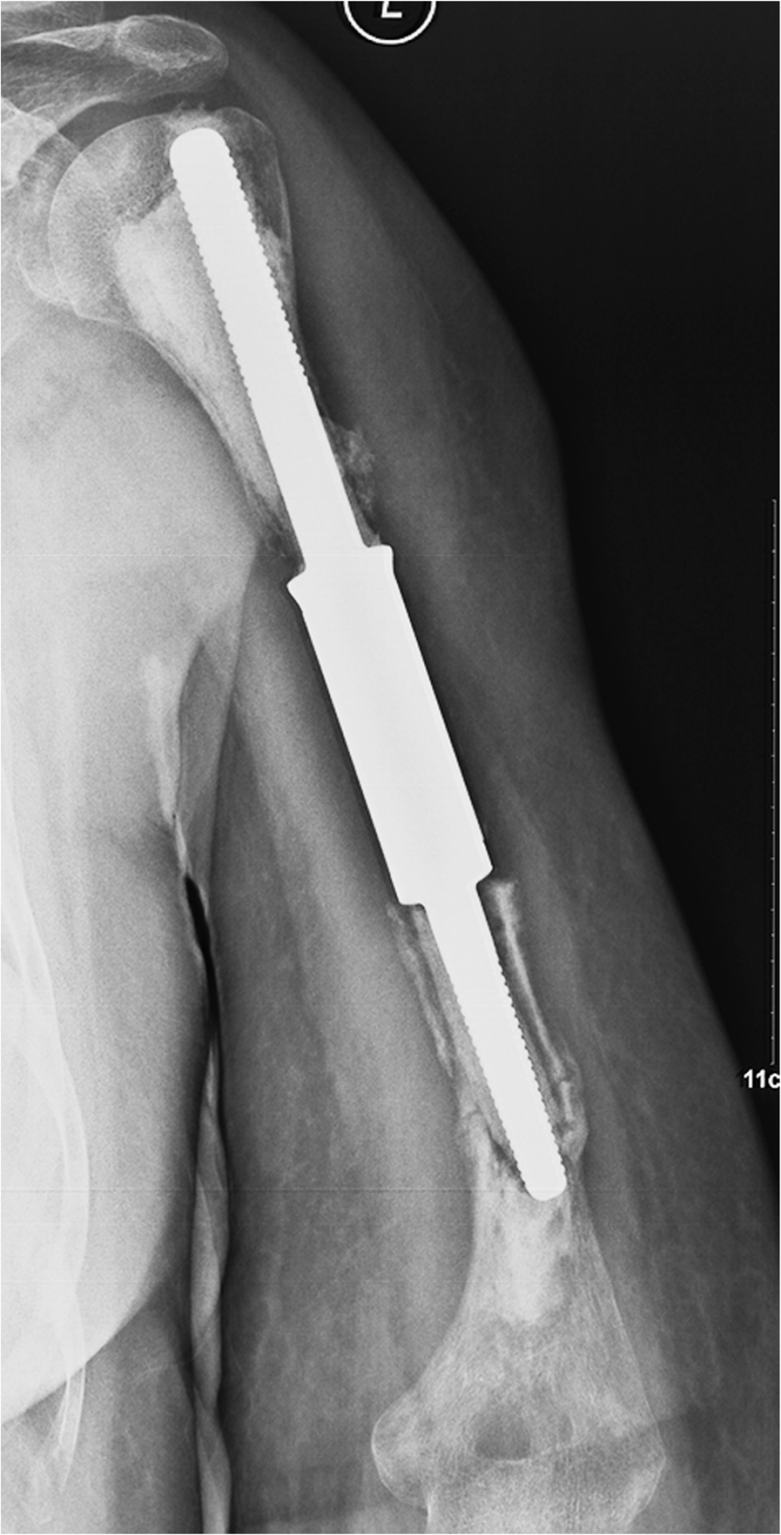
The postoperative radiograph taken 3 years after surgery shows loosening in the distal stem of the prosthesis caused by overuse; however, the function of this limb is still acceptable. The patient did not receive additional surgical management due to the presence of a brain metastasis
